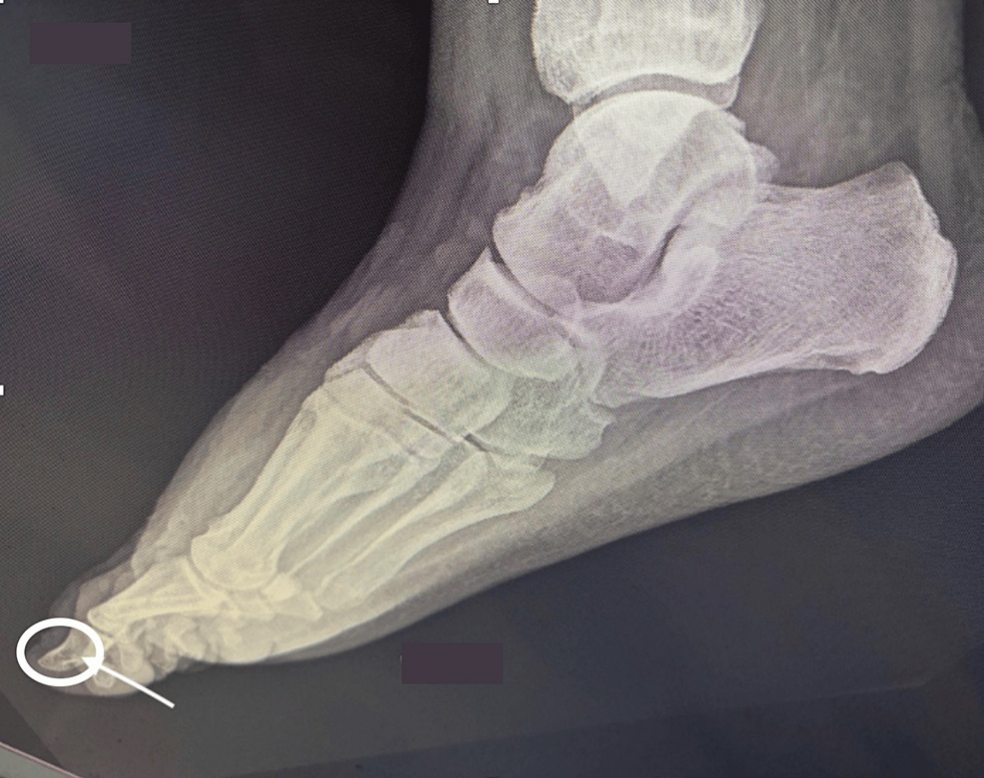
Lateral view of the right foot X-ray with a subtle air pocket in the distal aspect of the first phalanx Air pocket encircled. The distal aspect of the first phalanx is indicated by an arrow.
